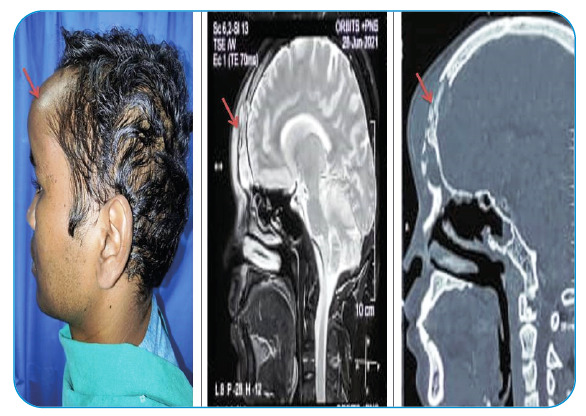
Images showing the swelling of the forehead and related computed tomography (CT) and contrast findings.
